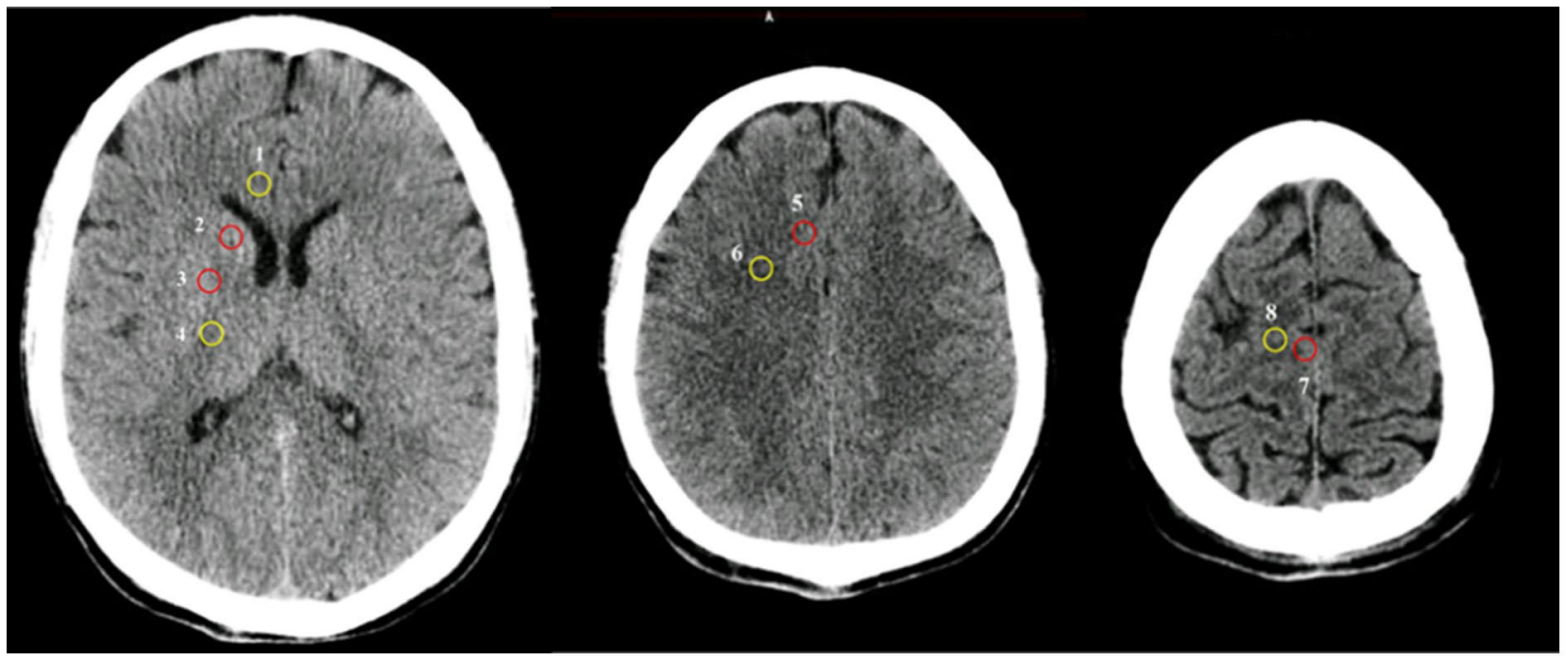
Circular regions of interest were placed bilaterally in the Cranial CT. 1 corpus callosum (CC), 2 caudate nucleus (CN), 3 putamen (PU), 4 posterior limb of internal capsule (PLIC), 5 cortex matter at the centrum semiovale level (MC1), 6 white matters at the centrum semiovale level (WM1), 7 cortexes at the high convexity level (MC2), 8 white matters at the high convexity level (WM2). Red circle: grey matter, yellow circle: white matter.
